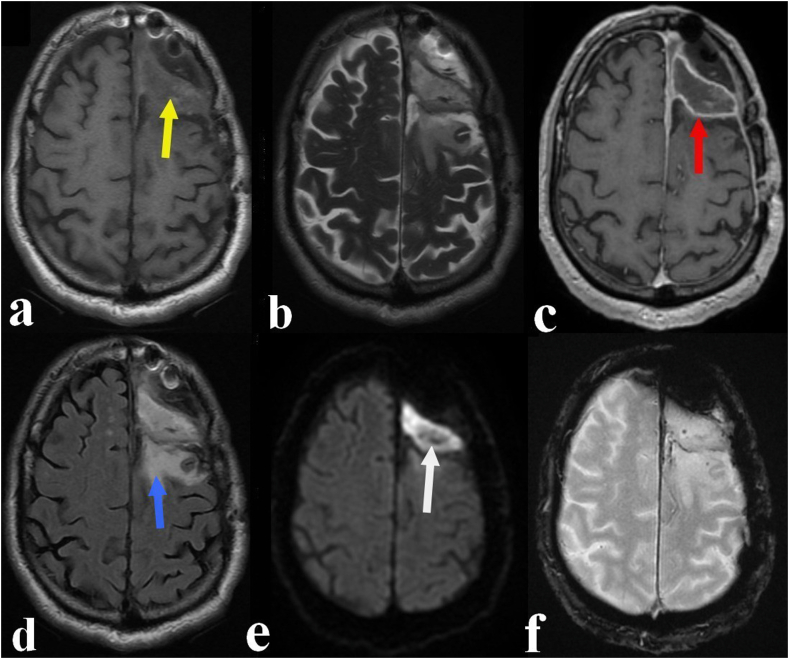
Axial brain enhanced MRI revealing a left frontal fluid collection at the site of the measuring 65 × 69 × 70 mm in diameter. This collection appeared in isosignal on T1-weighted sequence (a; yellow arrow) and discrete hypersignal on T2-weighted sequence (b) with heterogeneous and peripheral enhancement after gadolinium chelate injection (c; red arrow). On T2 FLAIR sequence (d; blue arrow), it was surrounded by a perilesional edema with a significant hypersignal on DWI image (e; white arrow). There was no bleeding on the gradient echo GRE sequence (f). (For interpretation of the references to colour in this figure legend, the reader is referred to the Web version of this article.)
